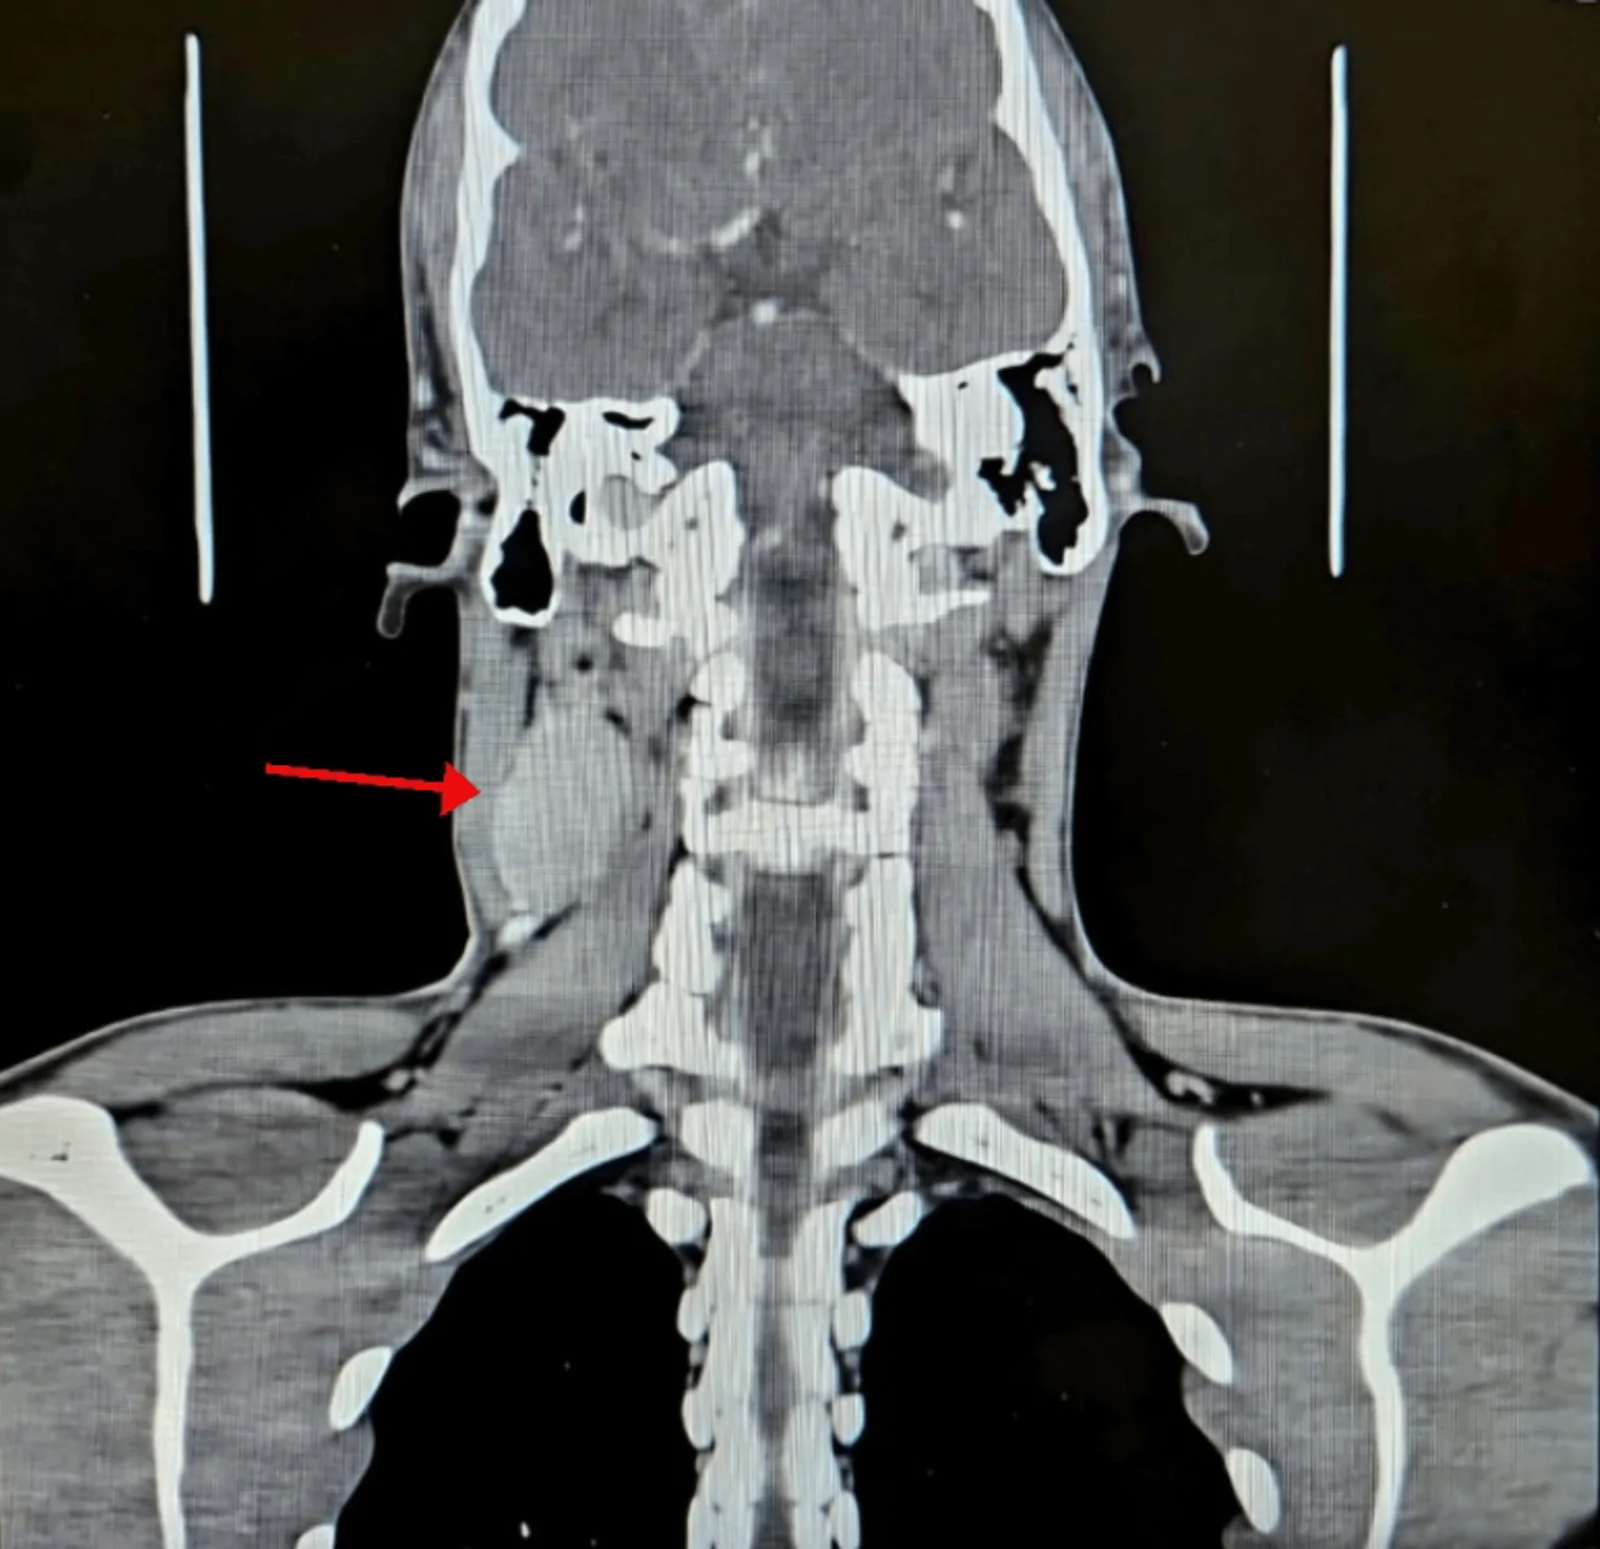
Coronal contrast-enhanced computed tomography (CT) of the neck and chest A bulky nodal mass and multiple confluent lymphadenopathies with suspicious features for secondary involvement are visualized, located in the right middle and lower jugular chain with caudal extension.
